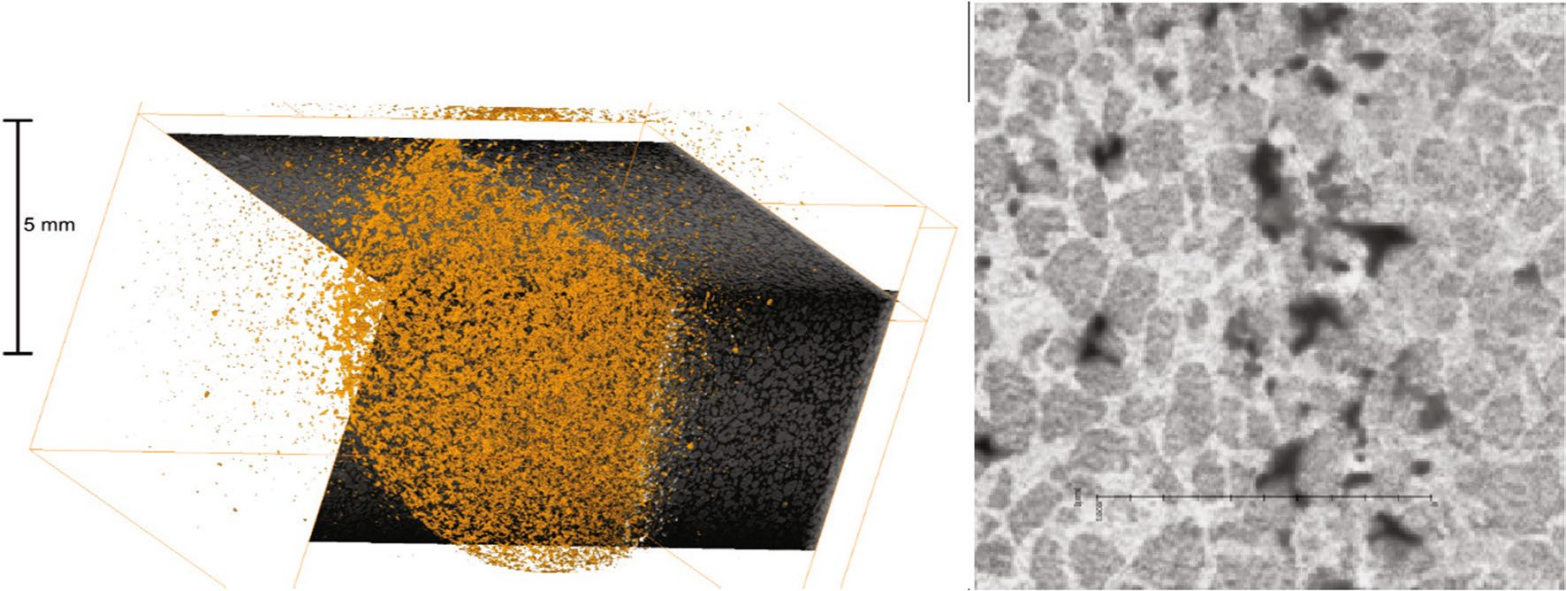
Left* 3D rendering of the segmented celestite grains (in *orange*) from the high resolution CT images of experiment Prec1. *Right* celestite precipitation zone with celestite appearing in *dark grey*, sand grains in *light grey* and pore space in *white* (horizontal length 1.5 mm).
